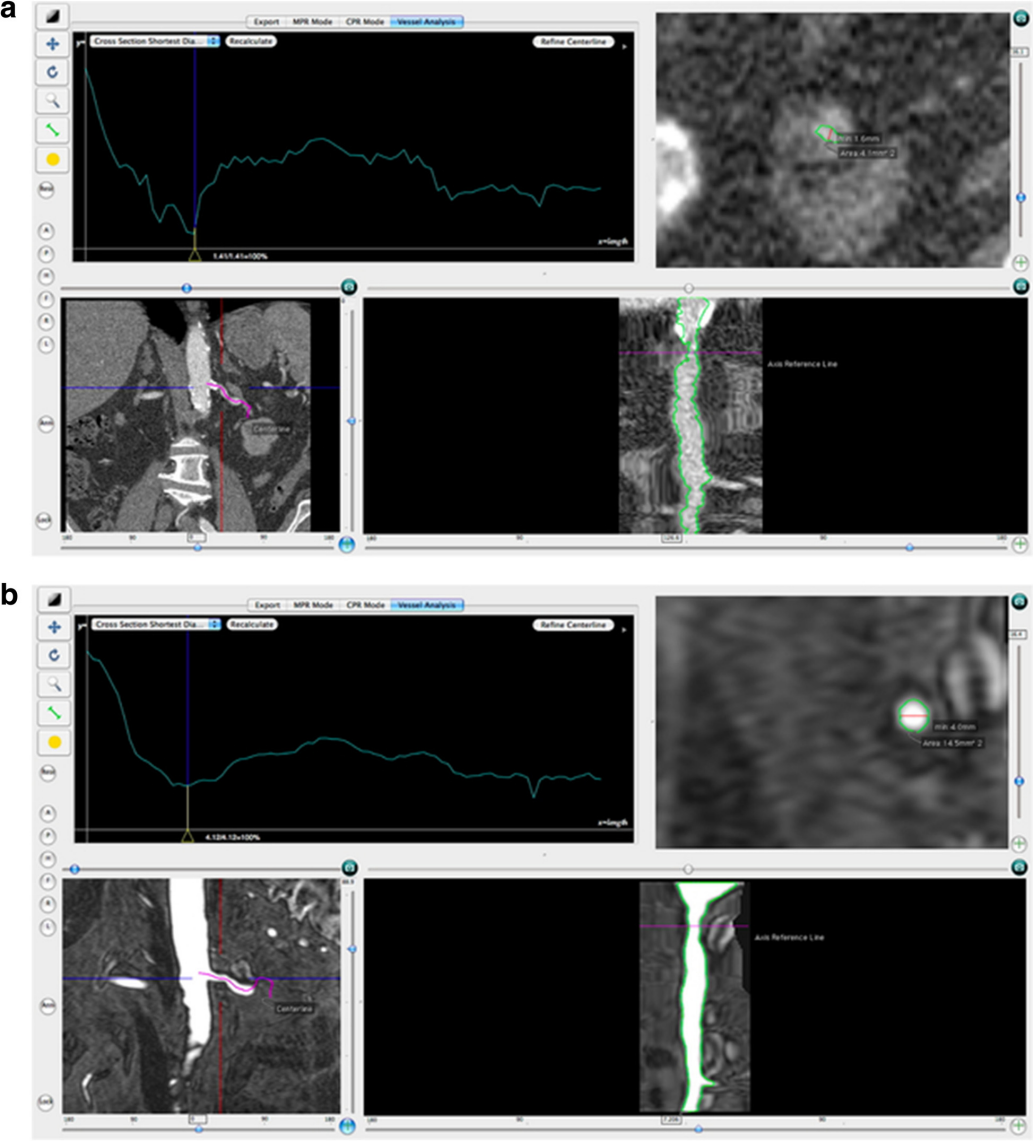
a, b. A view of the left renal artery in the OsiriX CTA plug-in from CT angiography (a) and MR angiography (b). In both images, the upper left part is a graph where the vertical axis represents the shortest cross-section diameter in the selected vessel and the horizontal axis represents the distance from the start of the vessel. The upper right part is a cross-section view of the marked position in the graph (in these cases the smallest minimum diameter), the lower left part is a coronal section showing the aorta, the renal arteries and surrounding tissues and the lower right part is the curved plane reformatted view of the chosen left renal vessel. Both modalities show the same stenosis, but it is more accentuated on the CT angiography
